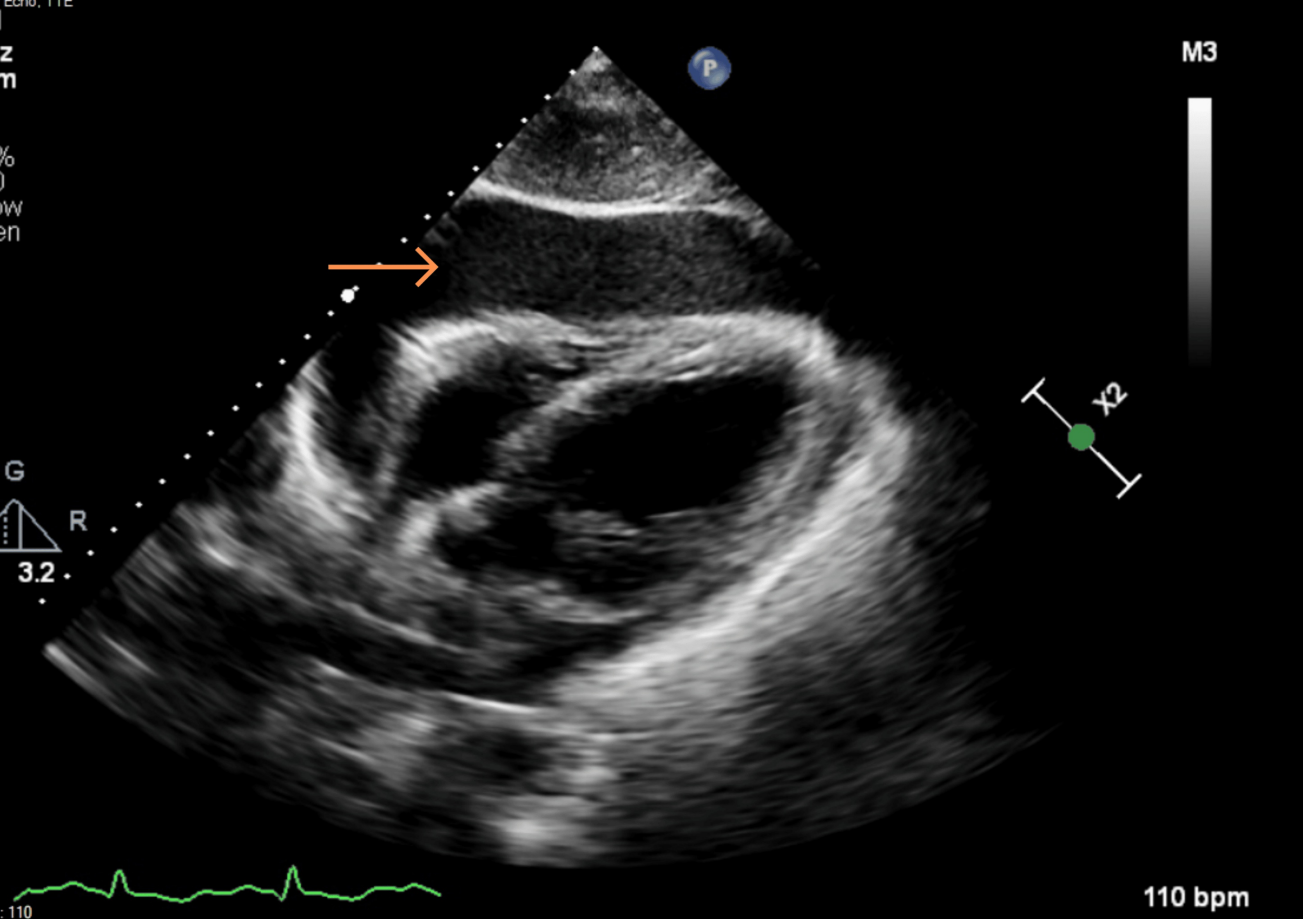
TTE subcostal window - pericardial effusion (orange arrow) TTE: Transthoracic echocardiogram
